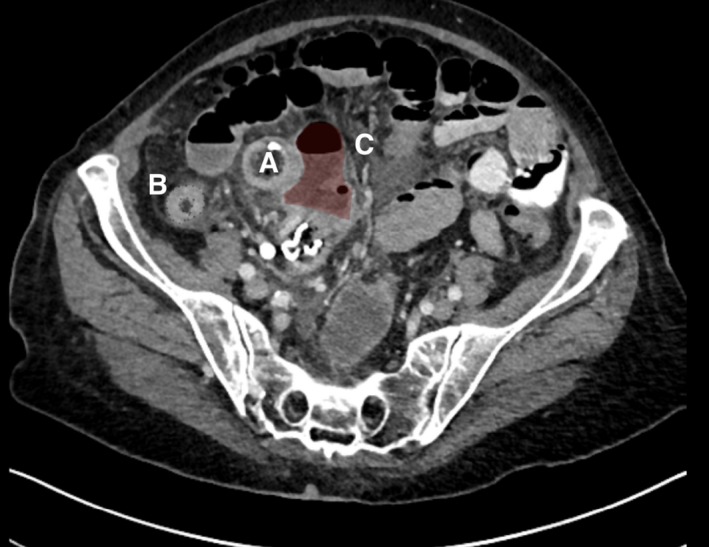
Axial abdominal CT with dilated distal ileum (A), thickened intestinal wall (B), and an interenteric abscess (reddish Zone ‐ C)
